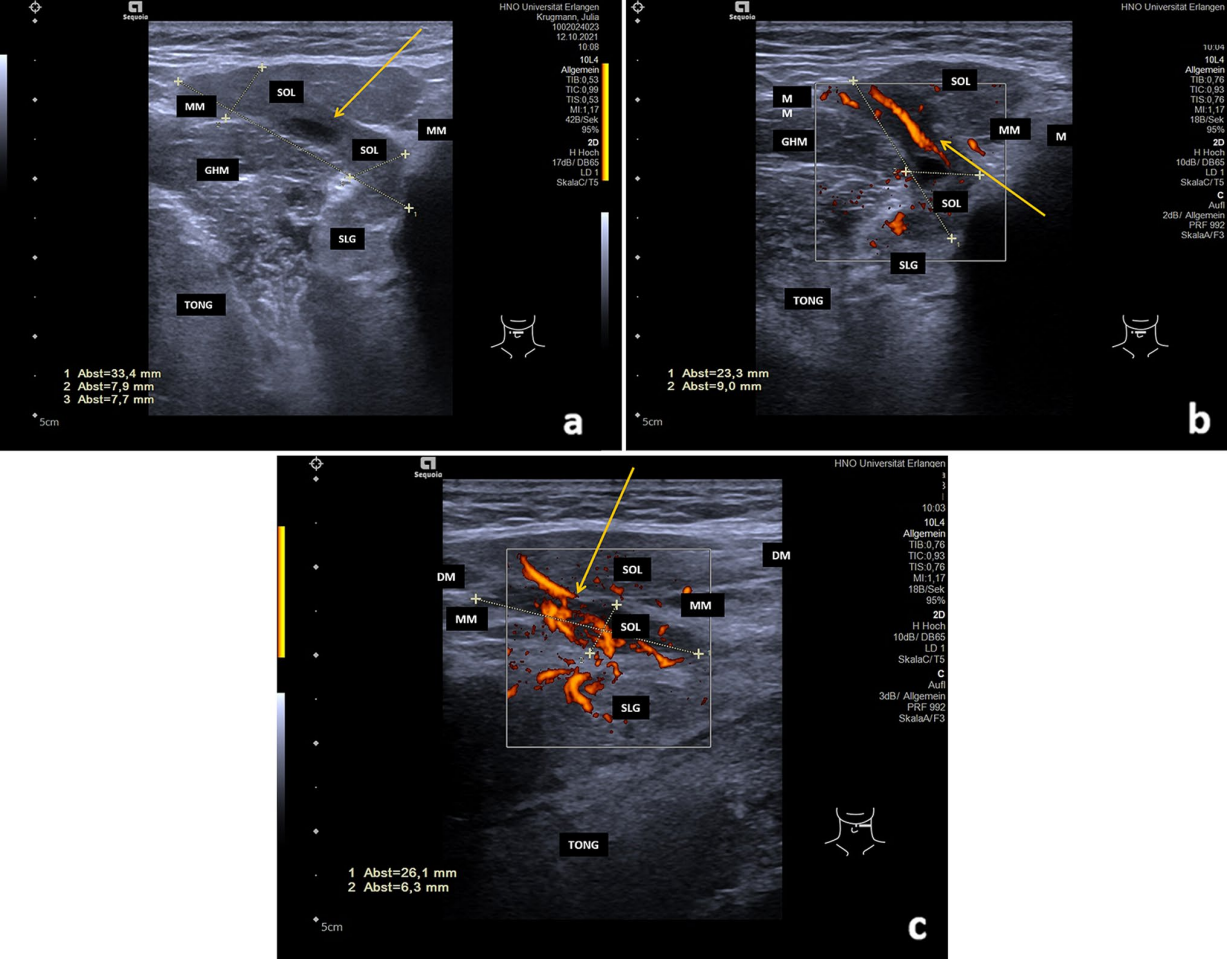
a–c Anterior transection view of the floor of the mouth native (a) and with low-flow doppler sonography (b) shows a PR in a 23 year old female patient (size 30 × 27x44mm): the moderate hypo-echoic and irregular shaped PR (SOL) is located anterior and deep to the SLG and partially above and partially below the MM in the anterior part of the floor of the mouth and is reaching to the submandibular space (a, orange arrow). The low-flow doppler sonography shows that the PR is perfused also in the region of the plunging through the hiatus of the MM (diameter 23.3 mm, orange arrow, b). Video 1 of the supplemental material is corresponding to this imaging. Lateral transection view (c) with low-flow doppler sonography shows that the PR (SOL) is partially perfused, in particular in the region of the plunging through the hiatus of the MM (diameter 26.1 mm) and is extending to the submandibular space (orange arrow, C). A puncture of the SOL revealed secretion characteristic for ranula. To exclude any combined pathology (e.g. PR and vascular malformation), MRI was also indicated. Video 2 of the supplemental material is corresponding to this imaging. *GHM* geniohyoid muscle, *DM* digastric muscle, *MM* mylohyoid muscle, *SOL* space occupying lesion, *SLG* sublingual gland, *SMG* submandibular gland, *TONG* tongue, *M* mandible
